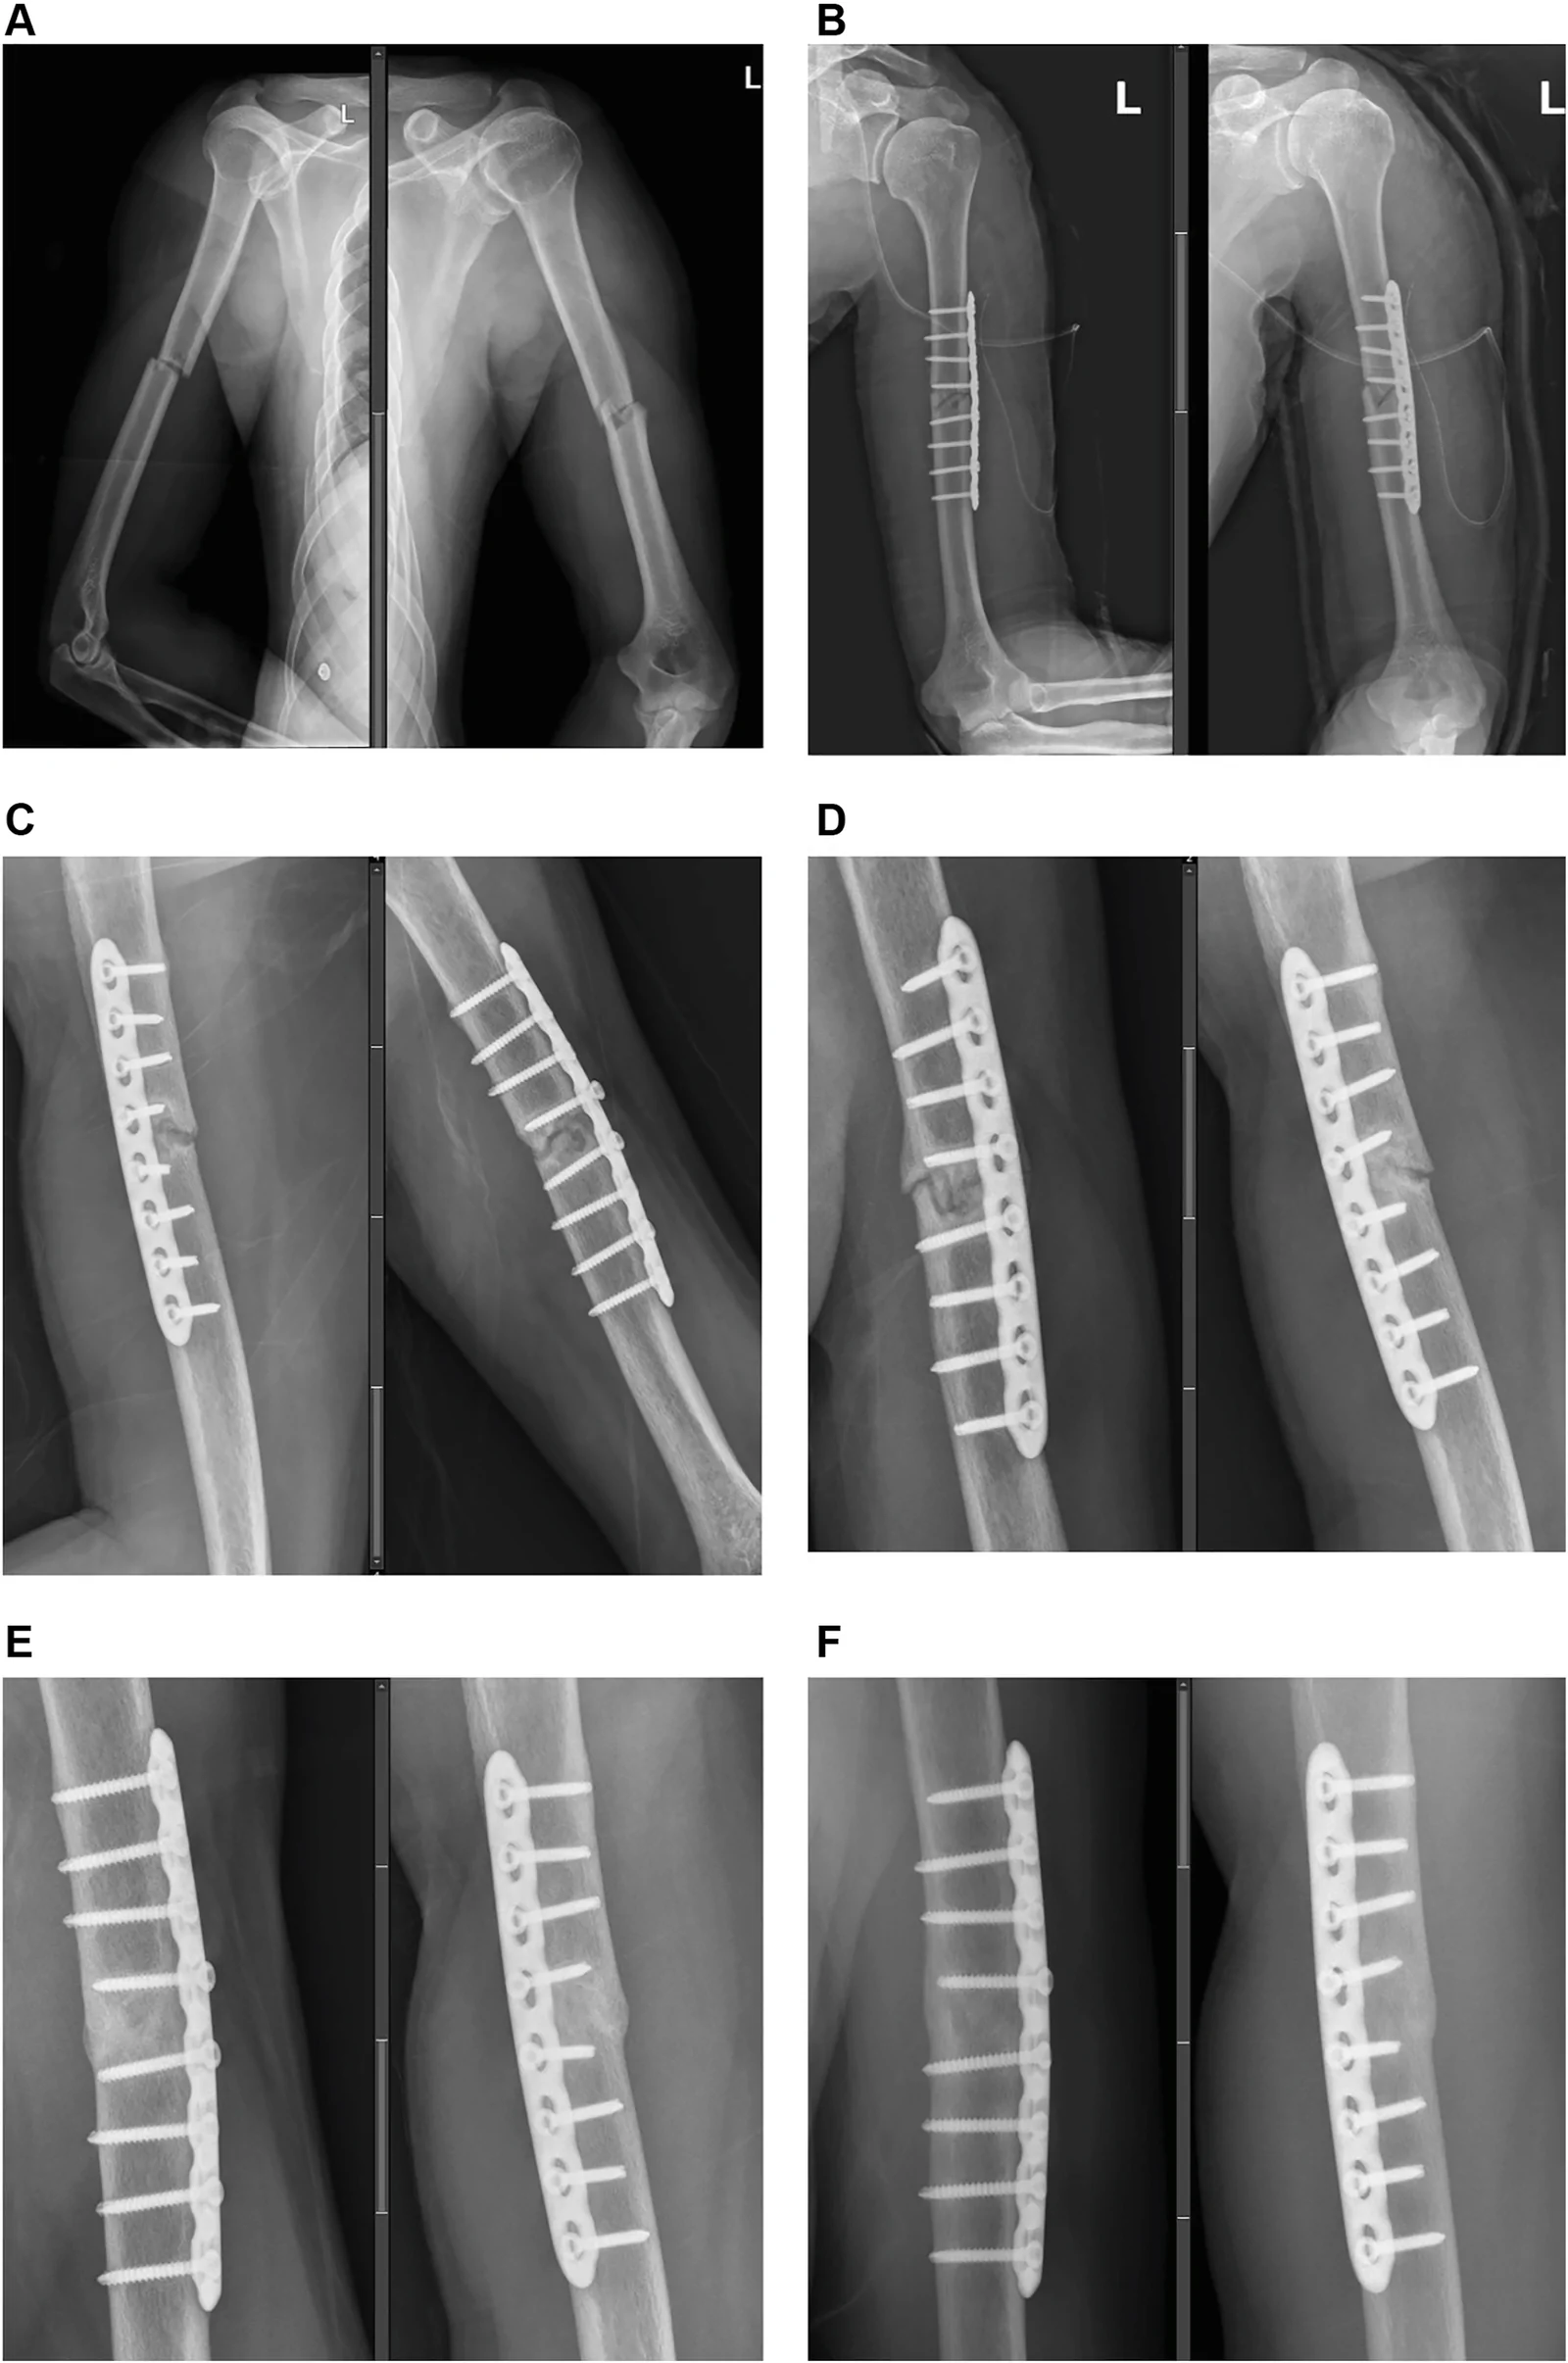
Serial radiographs of Case 2, a 60-year-old man with established delayed union and plate-related mechanical compromise after plate fixation for a left humeral shaft fracture. (A) Initial fracture radiograph. (B) Immediate post-operative radiograph after plate fixation. (C) Persistent delayed union at 12 months post-operatively. (D) Radiograph obtained 1 month after the third ESWT session showing early callus formation. (E) Progressive consolidation at 12 months after the third ESWT session. (F) Maintained union at the 2-year follow-up. *ESWT*, extracorporeal shock wave therapy.
